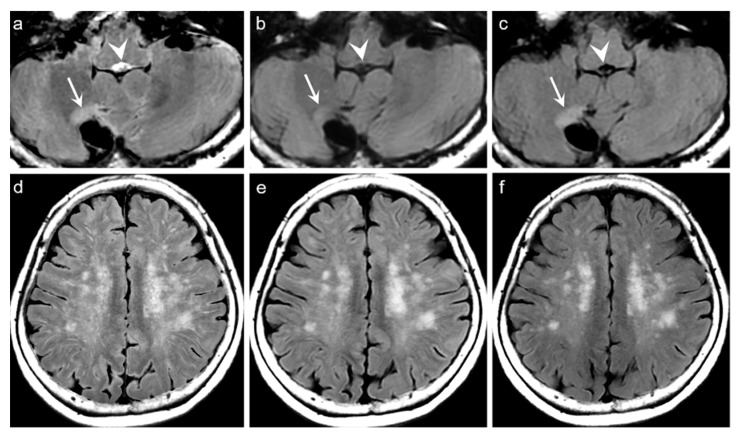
Representative case of lesion conspicuity on DL-FLAIR images. Focal marginal gliosis showing hyperintensity (arrows) is seen at the anterior aspect of the surgical cavity in the right cerebellum (a–c). The lesion is well delineated on the native synthetic FLAIR (a), DL-FLAIR (b), and conventional FLAIR images (c). However, the hyperintense lesion on the native synthetic FLAIR (arrow on a) is incompletely preserved on DL-FLAIR image (arrow on b), showing a decrease in the degree of its hyperintensity. Flow artifacts in the fourth ventricle on the native synthetic FLAIR (arrowhead on a) are successfully removed on DL-FLAIR image (arrowhead on b). Multiple FLAIR hyperintense lesions in both centrum semiovale, suggesting grade II small vessel disease on the native synthetic FLAIR (d) are well preserved on DL-FLAIR image (e). (f) Conventional FLAIR image is shown for comparison.
